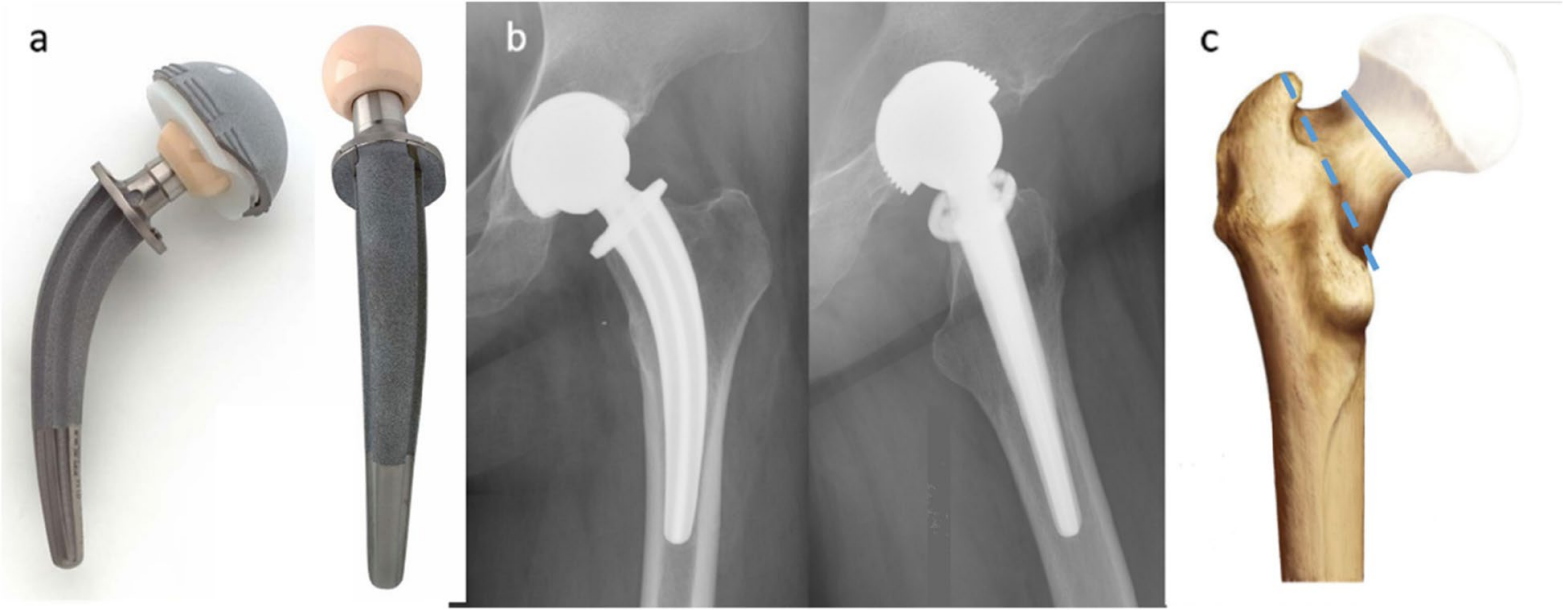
Characteristics of CFP prostheses. a. The geometry of the collum femoris-preserving stem (Waldemar-Link, Hamburg, Germany) can be characterized as a curvature design and self-anteversion. b. Postoperative anteroposterior and lateral radiographs of a 57-year-old female with left osteonecrosis of the femoral head. c. The osteotomy position of CFP stem is indicated by the solid line, and the traditional neck resection stem is indicated by the dotted line. The femoral neck between these two lines is preserved during hip arthroplasty when the CPF stem is implanted
